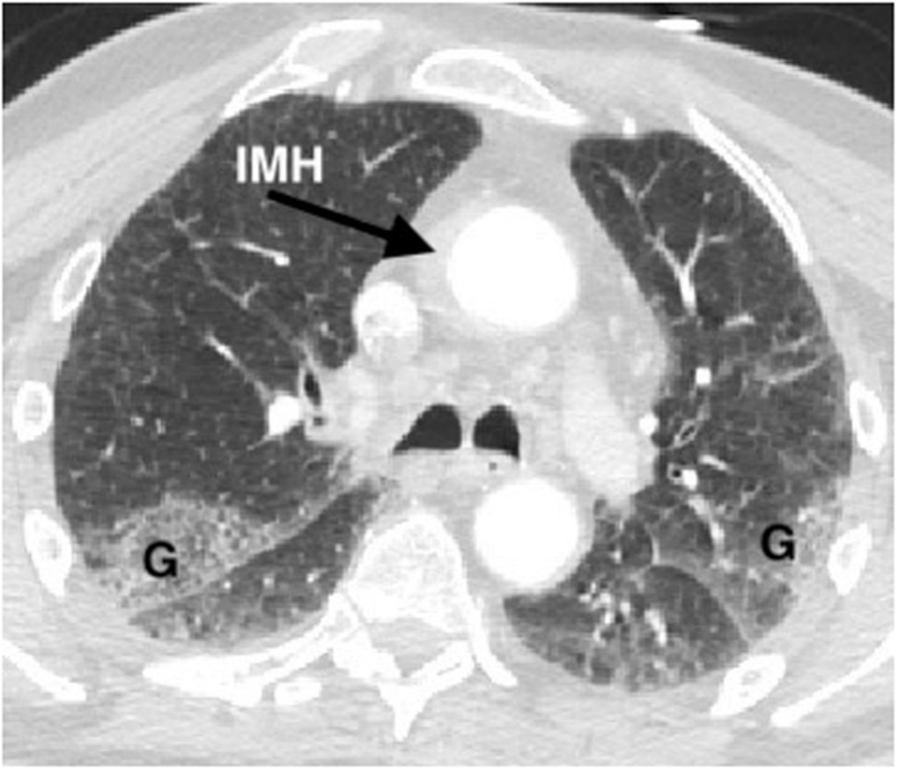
Computed Tomography. Computed tomography (CT) scan of the chest with contrast demonstrates an Intramural Hematoma (IMH) of the ascending thoracic aorta that extended from the aortic root to the proximal aortic arch. In addition, there were patchy peripheral ground-glass (G) opacities un the bilateral upper and lower lobes that have been associated with COVID-2019 infection
